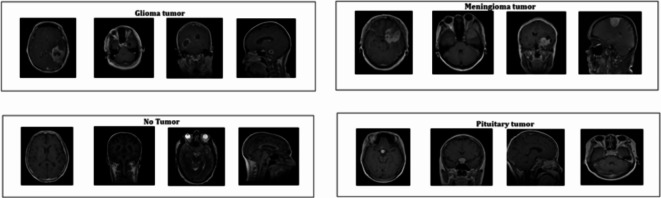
Sample MRI Images of Various Planes from the Dataset.
